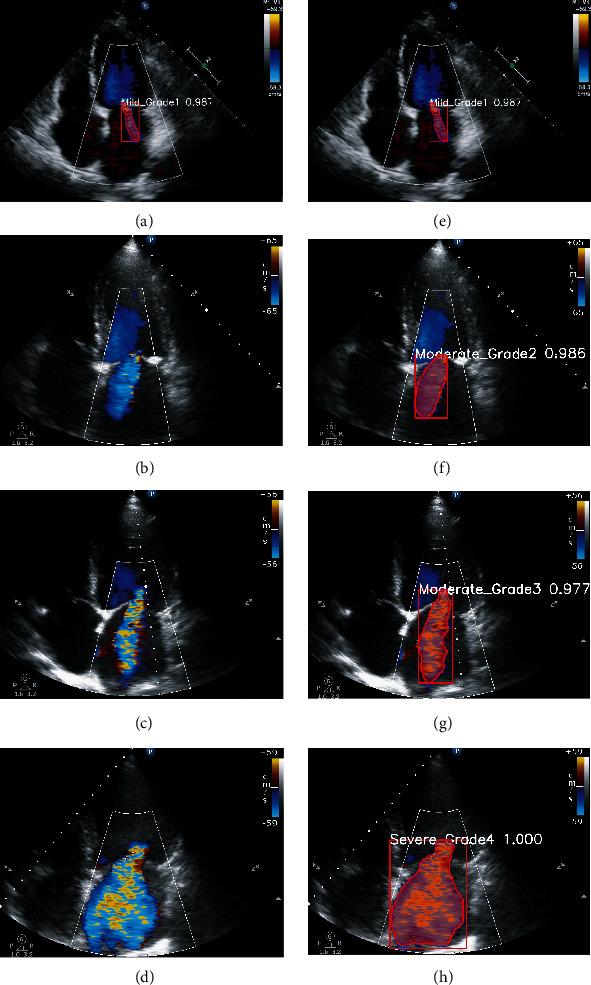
Test example of assessment of MR severity. (a–d) The MR images obtained by the quantitative evaluation methods of 2017 ASE guidelines. (e–h) The grading result obtained by the Mask R-CNN algorithm. The confident scores for the four cases were 0.987, 0.986, 0.997, and 1.000, respectively.
